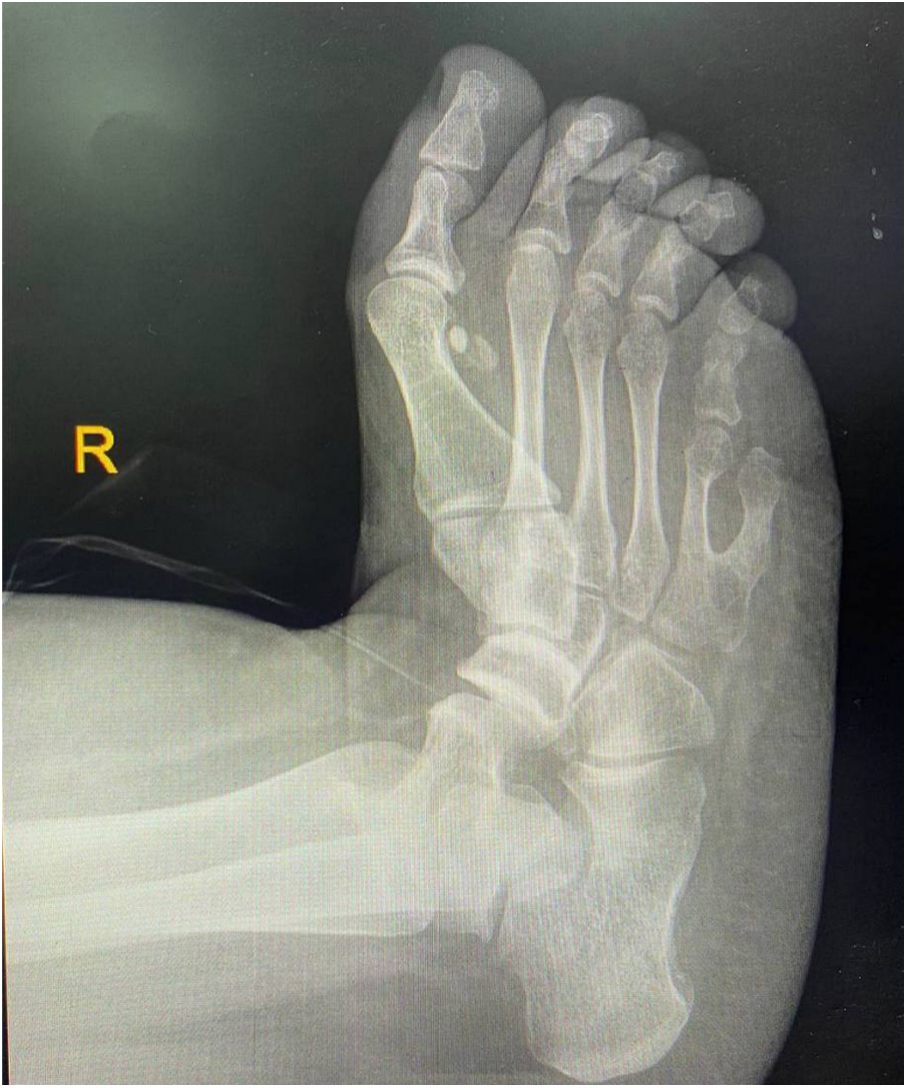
Radiograph demonstrating rocker bottom foot deformity.
